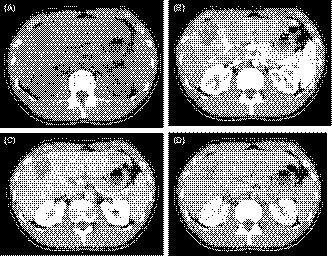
A, Plain computed tomography (CT) revealed multiple tumors with low‐density areas in both hepatic lobes. B‐D, Contrast‐enhanced CT showed the tumor with a slight circular enhancement in the early phase. The enhancement was prolonged to the delayed phase, up to 40 × 46 mm in size
